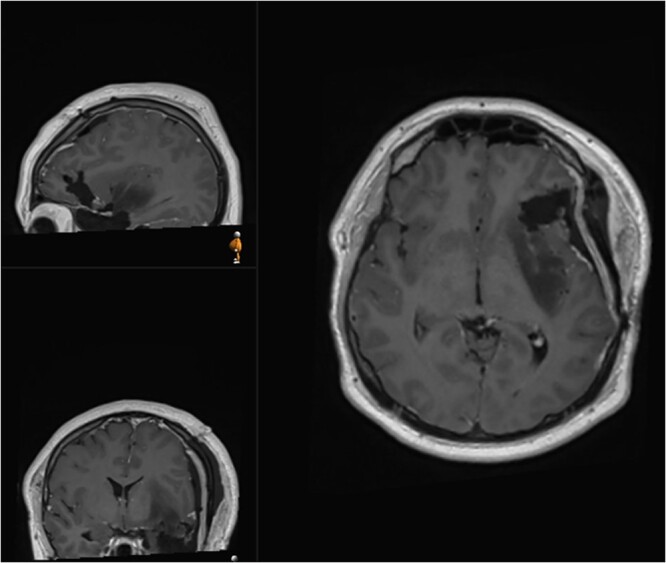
Postoperative (second surgery) MRI demonstrating residual tumour at the posterior margin of the insula.
